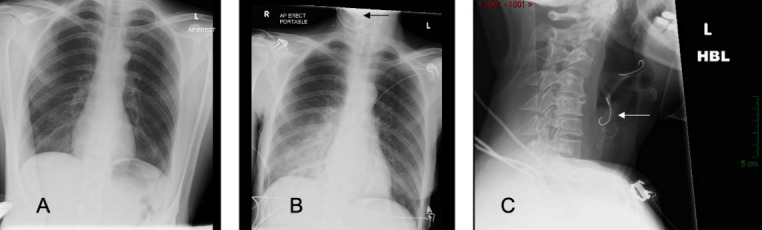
The X-rays in a patient who swallowed her denture with metal wires. On first attendance, the chest X-ray was clear (A); Chest X-ray on second admission included part of her lower neck which revealed denture wires in her pharynx (B), confirmed on soft tissue neck X-ray (C).
